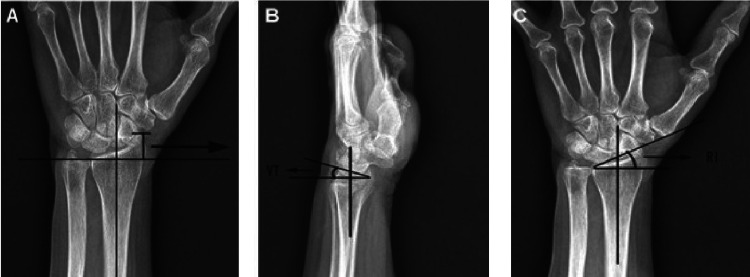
Methods of measuring radiographic parameters. A) radial height; B) volar tilt; C) radial inclination
